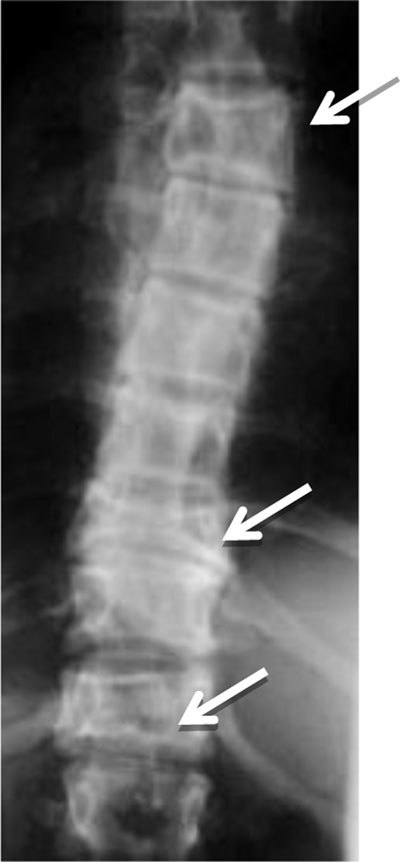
Polyostotic Fibrous Dysplasia. Radiograph shows collapse of multiple vertebral bodies (T6, T10, T12) with a ground glass matrix.
